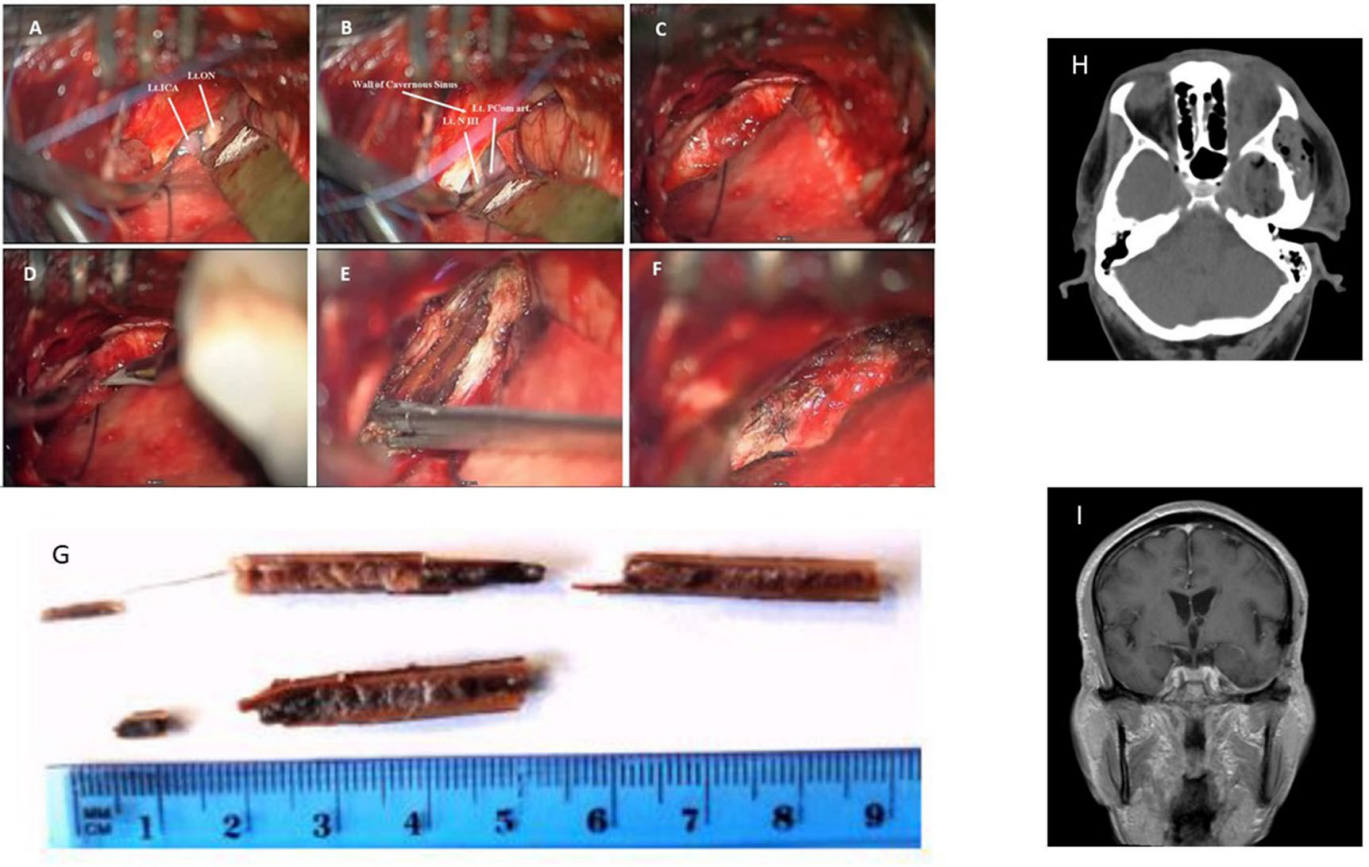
Intra-operative images: (A,B) Left CS lateral wall and adjacent non-injured anatomical structures: Lt. ICA—left Internal Carotid Artery, Lt. ON—left Optic Nerve, Lt. PCom—left Posterior Communicating Artery, Lt. NIII—left Oculomotor Nerve. (C) Superficial layer of CS lateral wall. (D) Opening of superficial layer of lateral wall of CS, and (E) Identification and removal of the FB. (F) Sutured superficial layer of CS lateral wall. (G) Photograph of the removed wooden FB fragments. (H) Post-op CT (I) Follow-up MRI scan a year after the injury.
